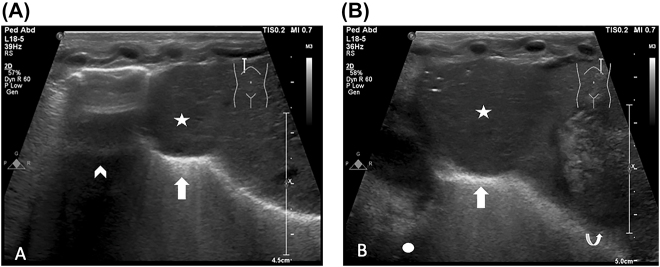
Selected longitudinal (sagittal) ultrasound images of right (A) and left (B) hemithoraces, demonstrating focal diaphragmatic eventration (arrows) with bulging of the dome of the liver into the eventration (stars). The right lower lobe of the lung (arrowhead), the heart (circle) and the stomach (curved arrow) are also visualised.
